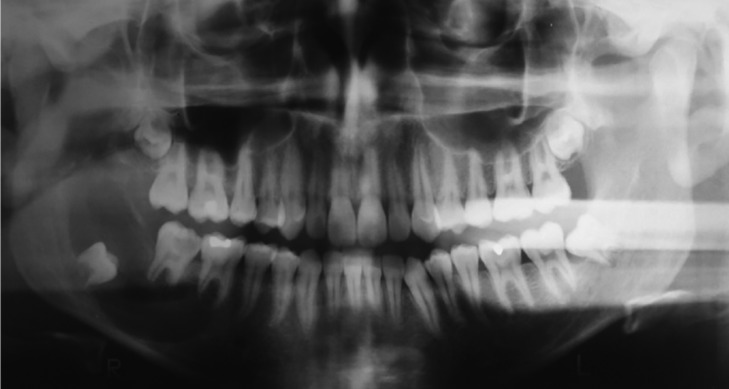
Panoramic radiography performed before marsupialization of the lesion in which the presence of the radiolucent, unilocular lesion associated to the tooth is observed 48.
